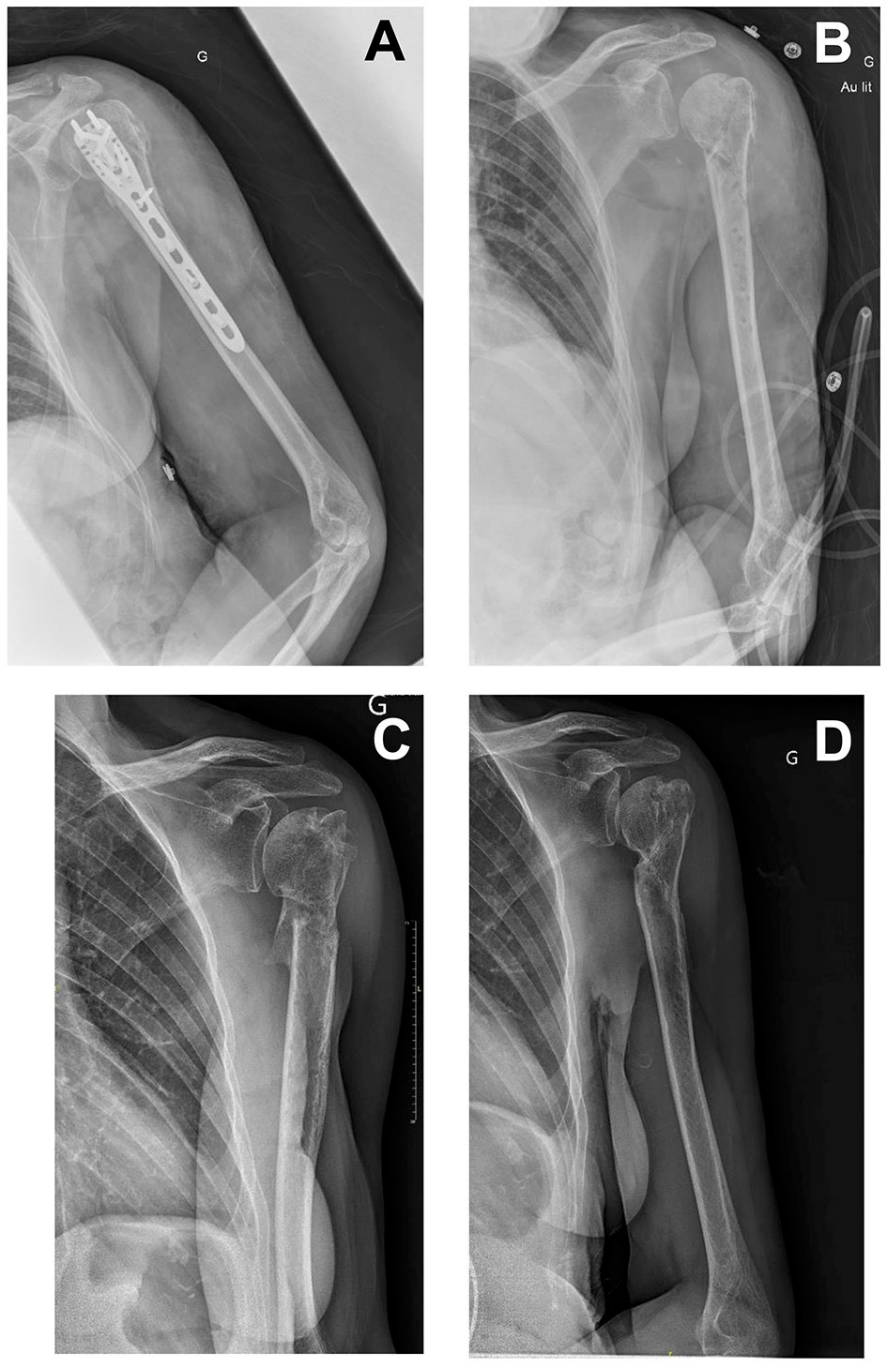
(A) An X-ray image of 2 days before debridement and implant removal surgery. (B) An X-ray image immediately after debridement and implant removal surgery. (C) An X-ray image of 6 months after surgery. (D) An X-ray image of 18 months after surgery.
